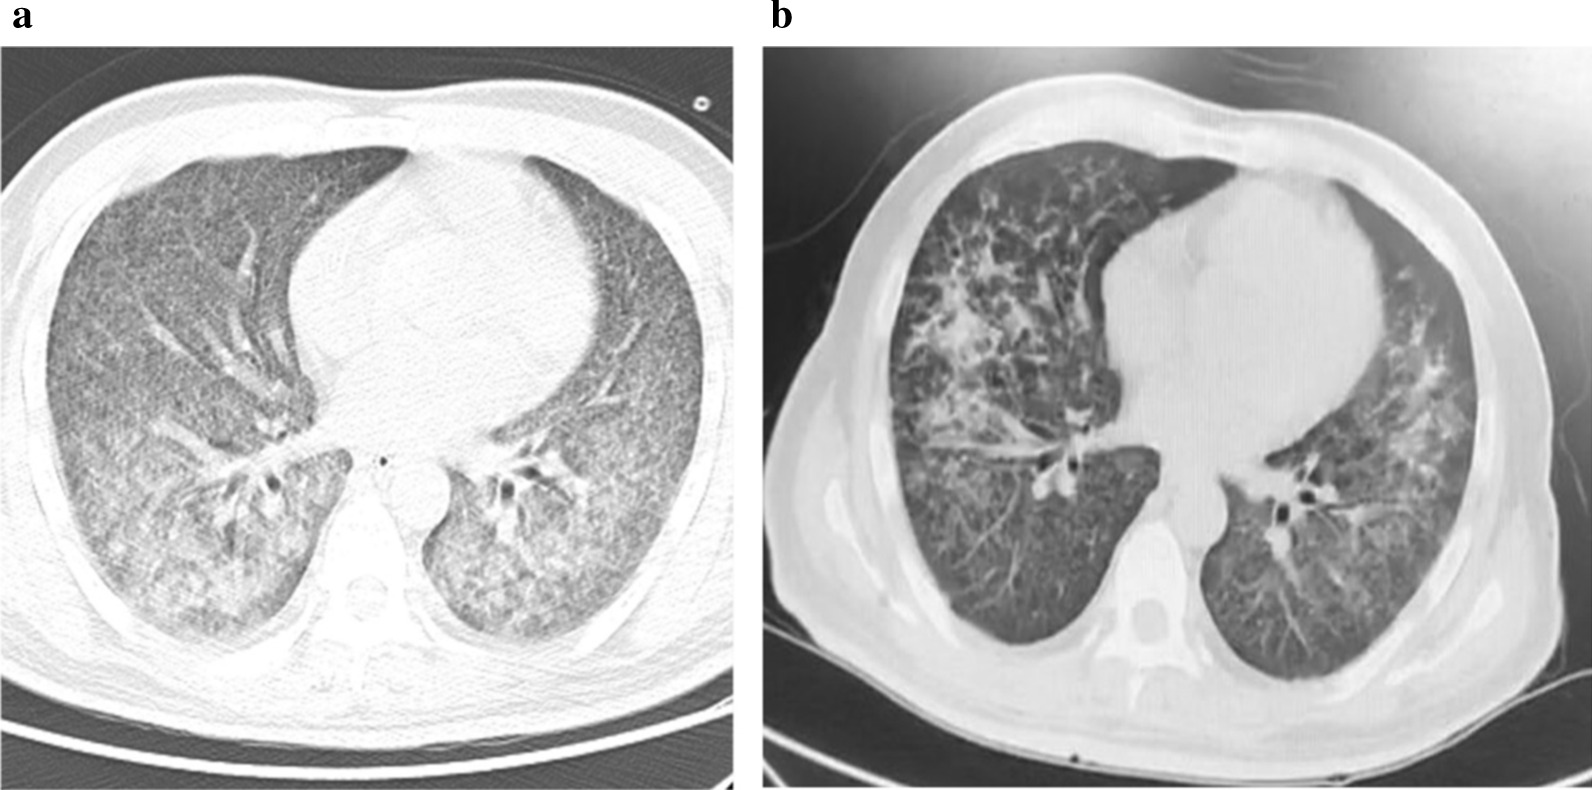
Chest computed tomography images after treatment. a. Chest computed tomography after 14 days of treatment. b. Chest computed tomography after 21 days of treatment
